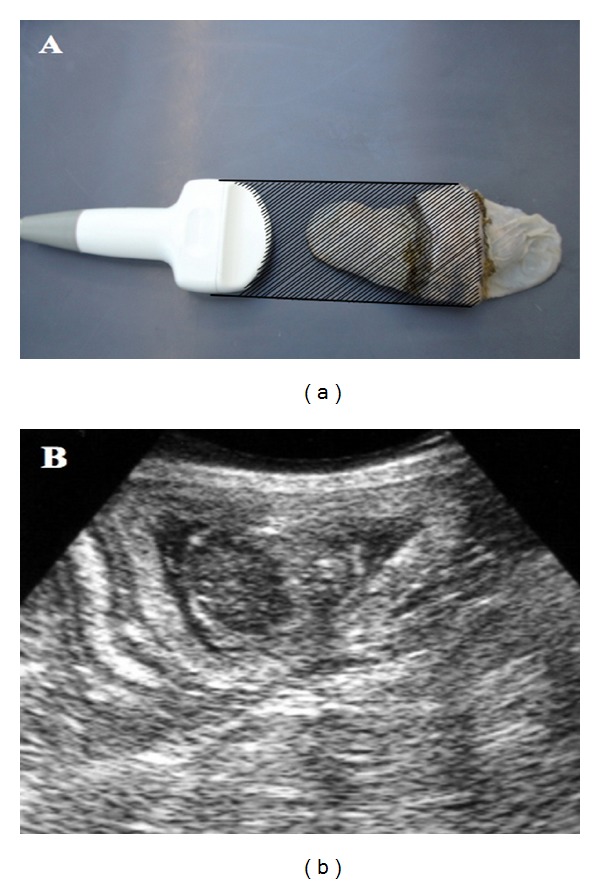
Planimetric image of the ultrasound beam's action angle in the placenta ring. The hachured area represents the angle (approximately 90°) of the beam's incidence on the placenta ring pars intermedia, the point where thickness measurements were performed (a). (b) Corresponding ultrasound image.
